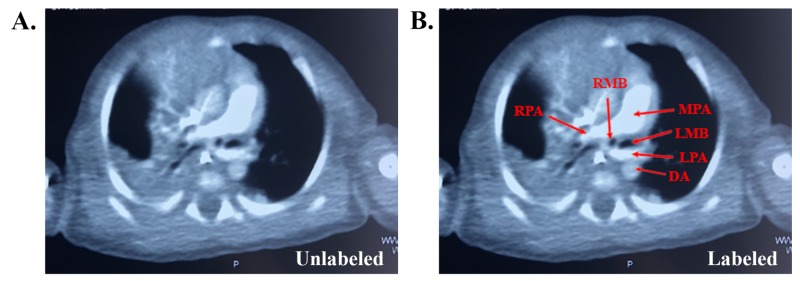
Thoracic CT scan showing developmental retro-tracheal malformation of the left main pulmonary artery originating from the right with compression of the origin of the right main bronchus (unlabeled in A and labeled in B). DA, descending aorta; LMB, left main bronchus; RMB, right main bronchus; RPA, right pulmonary artery; LPA, left pulmonary artery; MPA, main pulmonary artery
